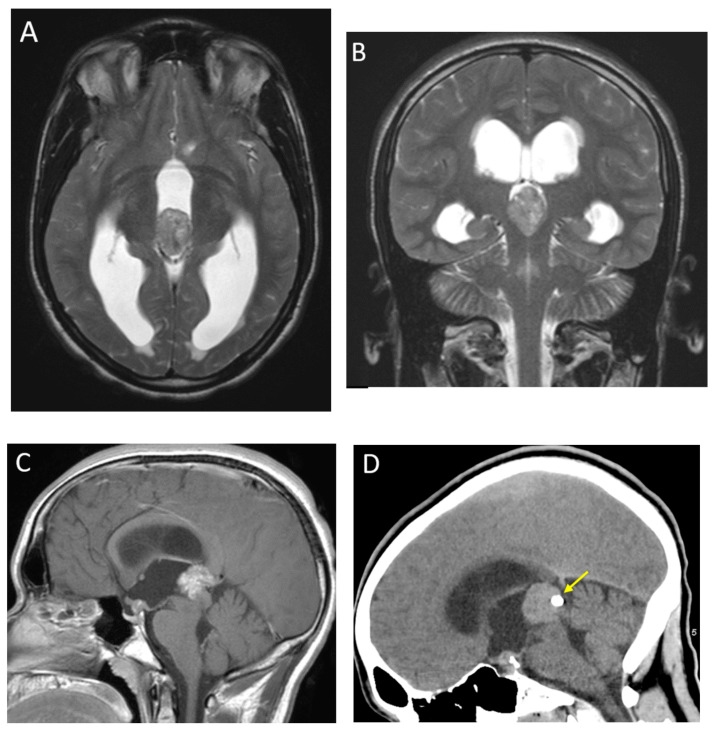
A 19-month-old male with with germ cell tumor and synchronous involvement of the pineal and suprasellar regions. Axial and coronal T2-weighted (A,B) images of the pineal region demonstrate heterogeneously T2-isointense mass with obstructive hydrocephalus. Sagittal post-contrast T1-weighted image (C) demonstrates heterogenous enhancement of the pineal region mass with obstruction of the cerebral aqueduct, and also a smaller, similar-appearing mass, involving the pituitary infundibulum (arrowhead). Sagittal non-contrast CT image (D) of the same patient demonstrates a rounded pineal calcification ‘engulfed’ by the mass (arrow), which can help to distinguish germ cell tumors from pineoblastomas.
